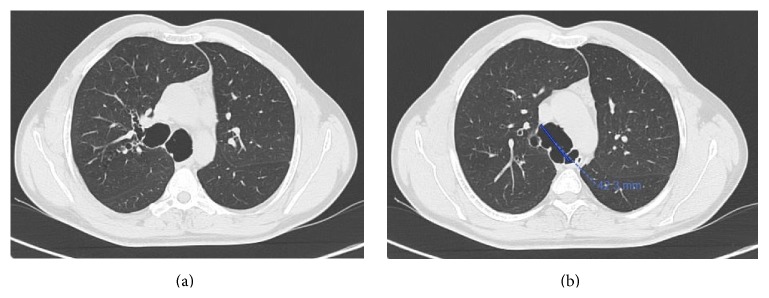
HRCT showing dilated trachea and the main bronchi.
